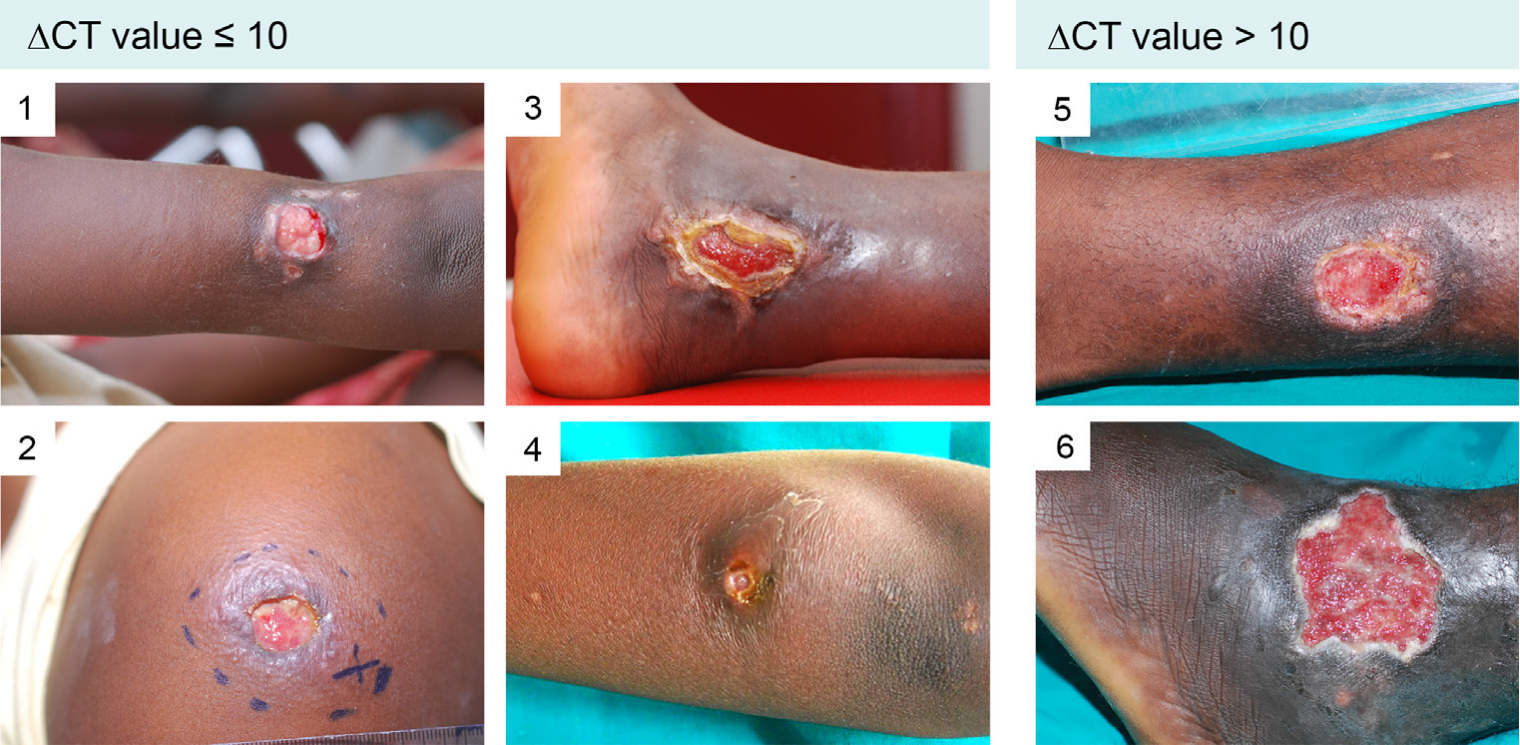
Examples of lesions presenting with both IS2404 qPCR positive and negative swabs. Lesions of six laboratory confirmed BU patients are shown, whereof four (# 1–4) presented with a ∆CT value ≤ 10, indicating a minimal to medium heterogeneity and two (# 5 and 6) presented with a ∆CT value > 10, indicative for a maximum heterogeneity. From each lesion four swabs have been taken in a clockwise manner and IS2404 qPCR has been performed.
